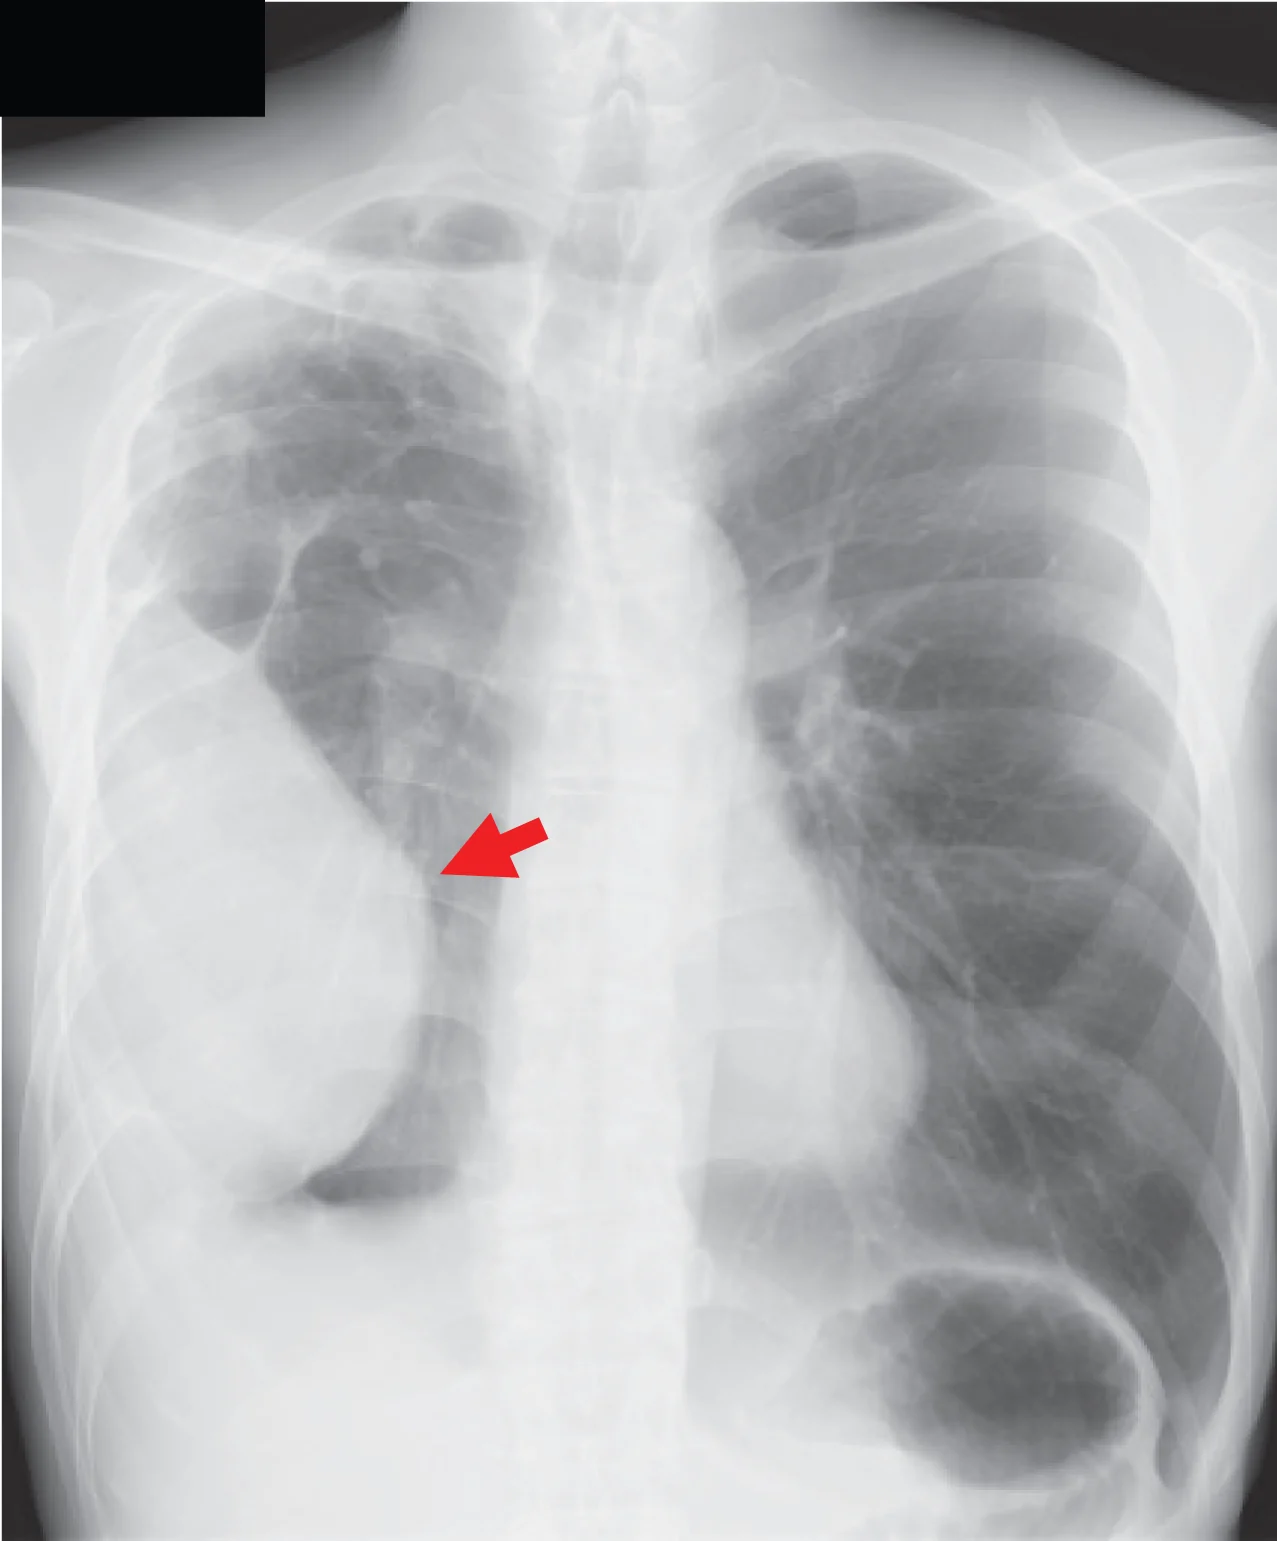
Chest radiograph findings. A chest radiograph showed a mass shadow in the right middle and lower lung fields (arrow).
